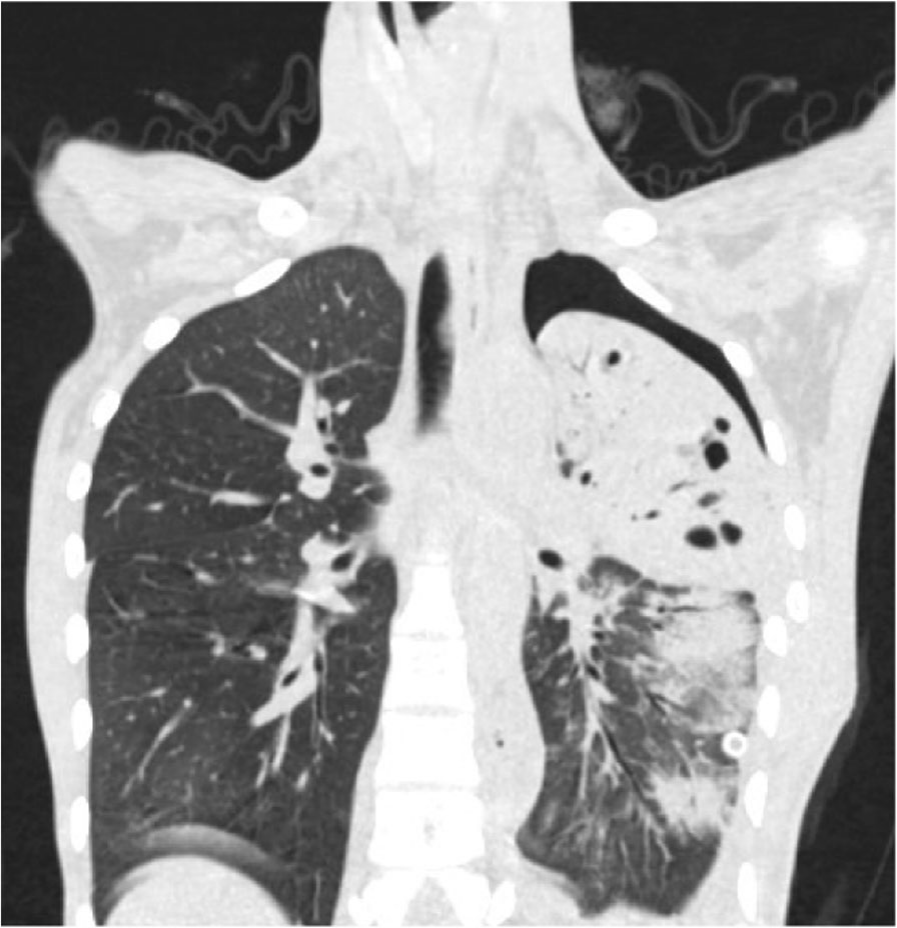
Computed tomography image 1 week after operation: substantial consolidation and several cystic lesions of various sizes
